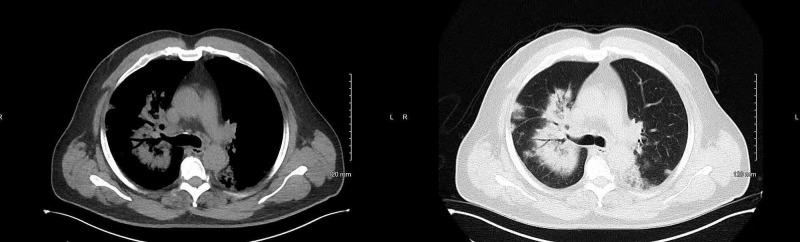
CT chest showing extensive bilateral alveolar infiltrates CT: computed tomography
